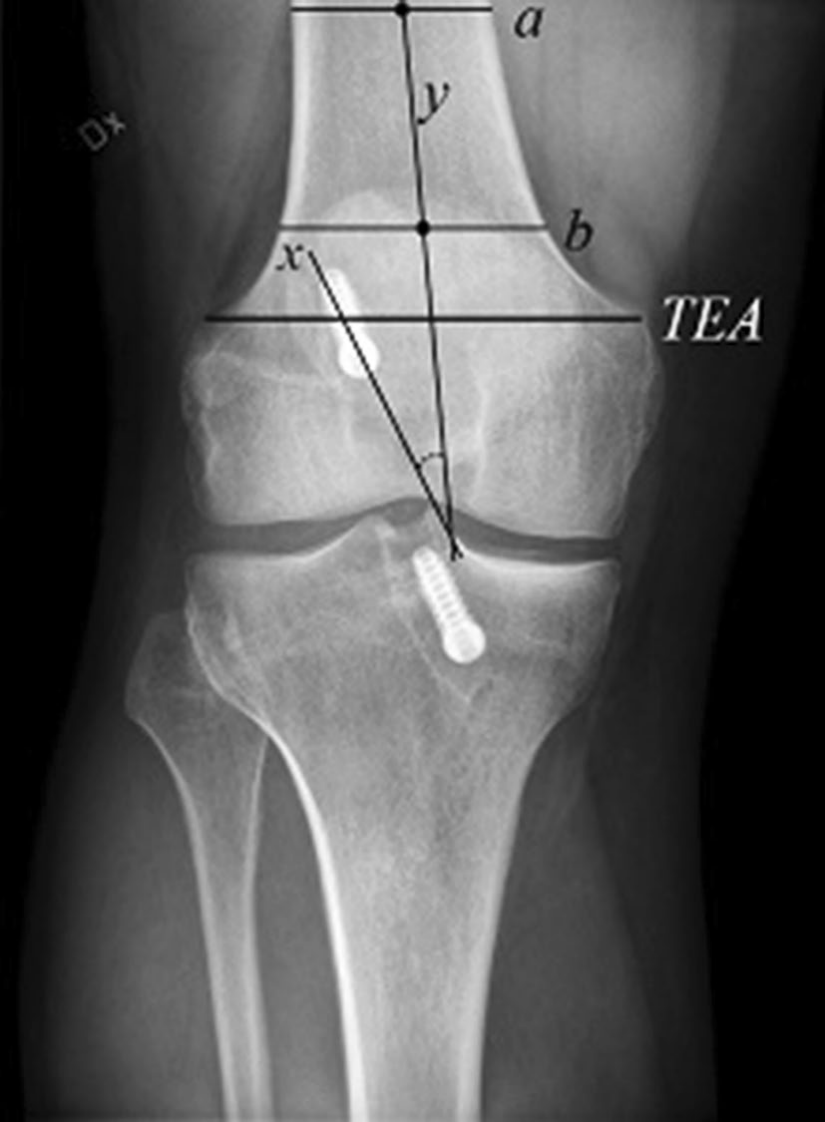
A radiograph showing the method used to measure the femoral tunnel angle formed by the femoral tunnel and the long axis of the femur in posteroanterior radiographs. The most proximal portion of the femoral epicondyle was bisected by a line forming the transepicondylar axis (TEA). At the most proximal part of the femur, visualized on the radiograph, a line was drawn along the width of the diaphysis and parallel to the TEA (a). Distal to this line, at a distance from line a that corresponds to half the length of the TEA, another parallel line was drawn (b). The midpoint of lines a and b was crossed by a line parallel to the axis of the diaphysis. The femoral tunnel was visualized and a line was drawn through its axis (*x*). The angle formed between this line and line y was defined as the femoral tunnel angle
